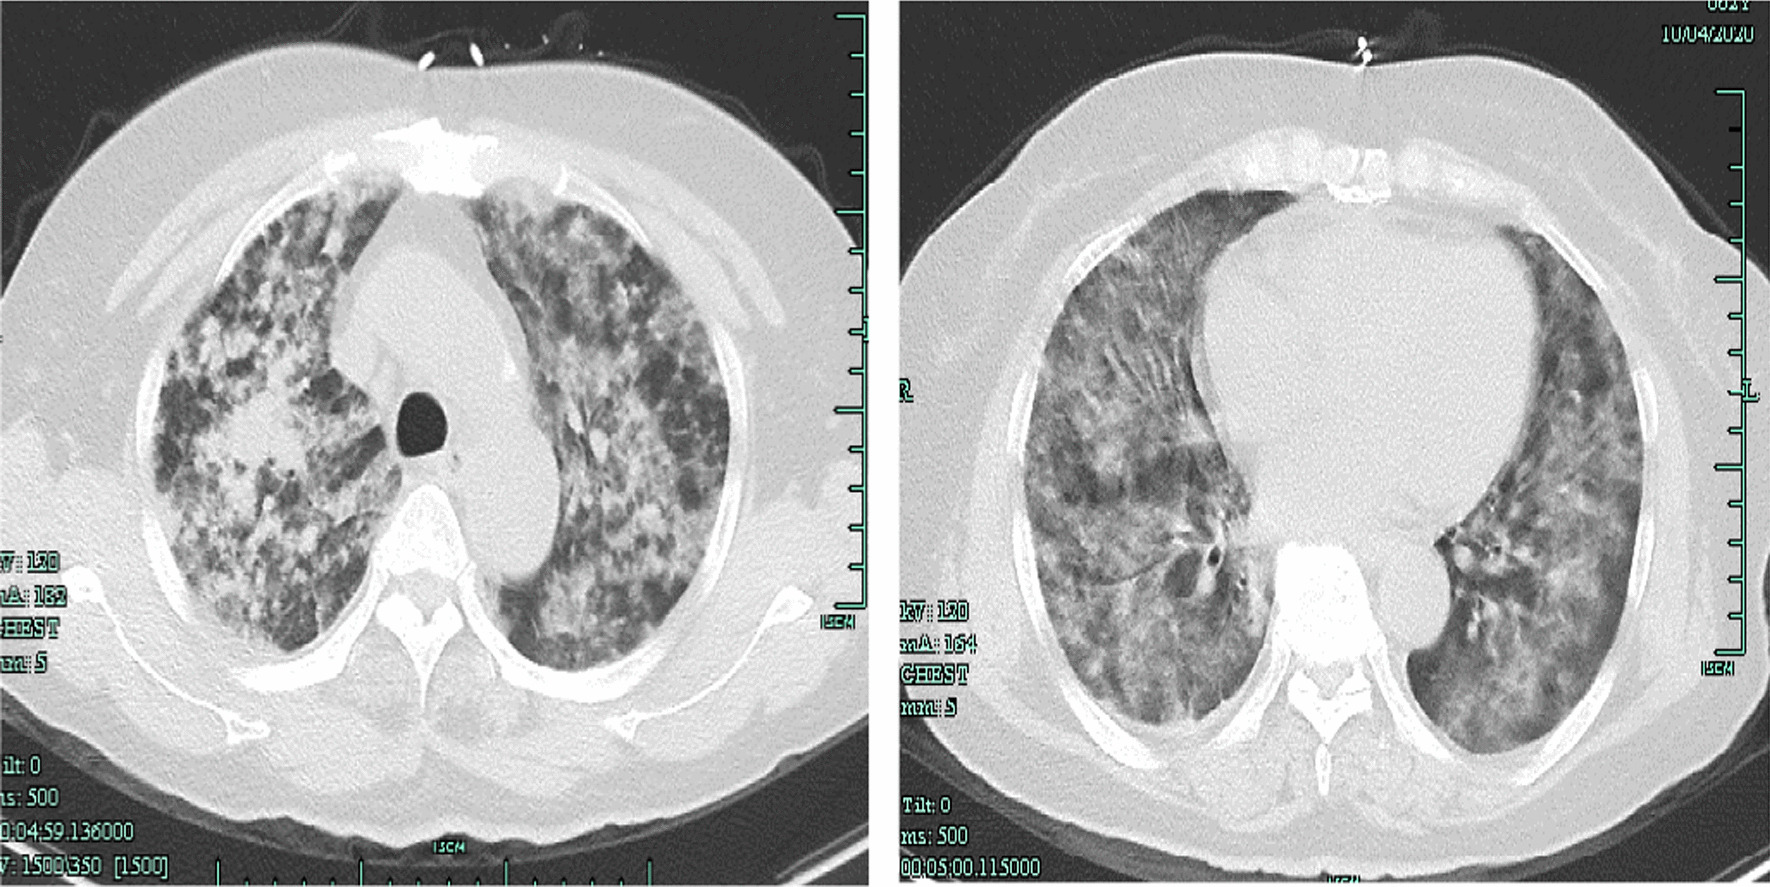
Chest CT showing diffuse alveolar infiltrates with acute respiratory failure on admission
